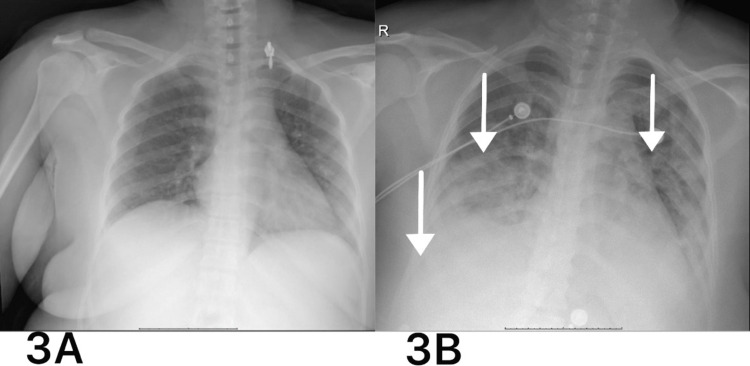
Chest X-ray 3A: Initial chest X-ray was grossly unremarkable. 3B: Repeat imaging was abnormal with evidence of bilateral hazy appearance involving the mid and lower lungs and blunting of the right costophrenic angle.
